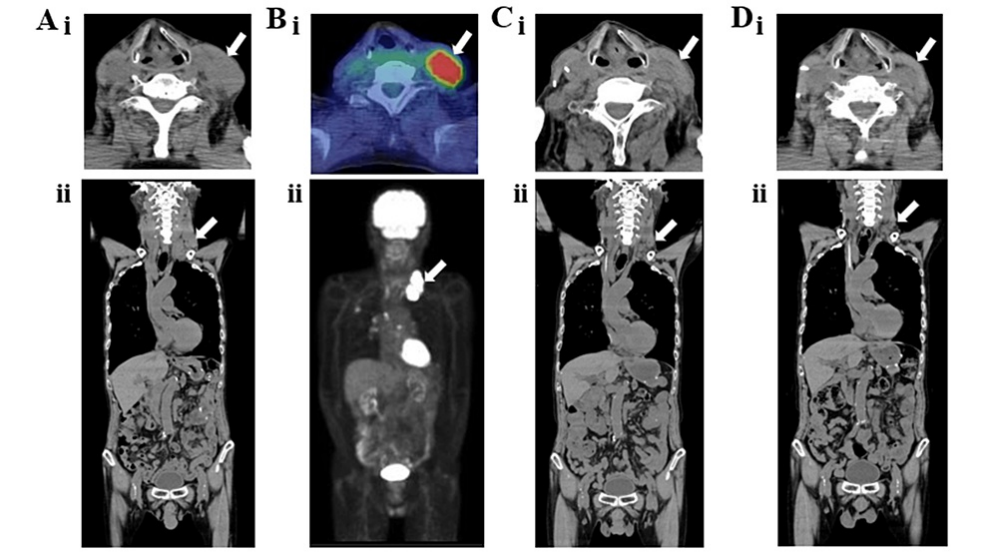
Radiological findings in Case two. (A) CT findings on June 23, 2022. (i) Fifth cervical vertebrae finding and (ii) systemic finding. (B) PET-CT findings on July 20, 2022. (i) Fifth cervical vertebrae finding and (ii) systemic finding. (C) CT findings on September 22, 2022. (i) Fifth cervical vertebrae finding and (ii) systemic finding. (D) CT findings on November 2, 2022. (i) Fifth cervical vertebrae finding and (ii) systemic finding. Arrows indicate left cervical lymphadenopathy CT: computed tomography; PET-CT: positron emission tomography-computed tomography
